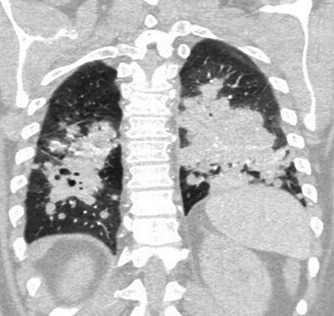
Coronal CT angiogram of the chest on admission. Widespread nodular consolidation through both lung fields, which has a cavitary component in the right lower lobe.
